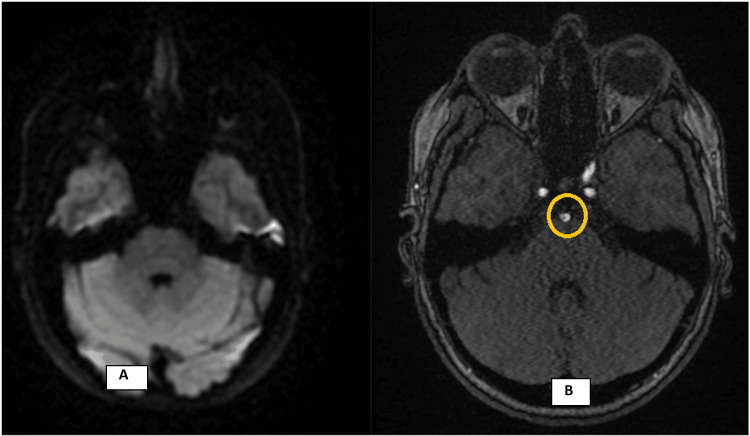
(A) MRI DWI b1000 image showing no diffusion changes in the posterior fossa; (B) TOF-MRA demonstrating a small central signal void in the mid-basilar artery, corresponding to the site of the hyperdensity previously seen on CT. TOF-MRA, time-of-flight magnetic resonance angiography; MRI DWI, magnetic resonance imaging with diffusion-weighted imaging
